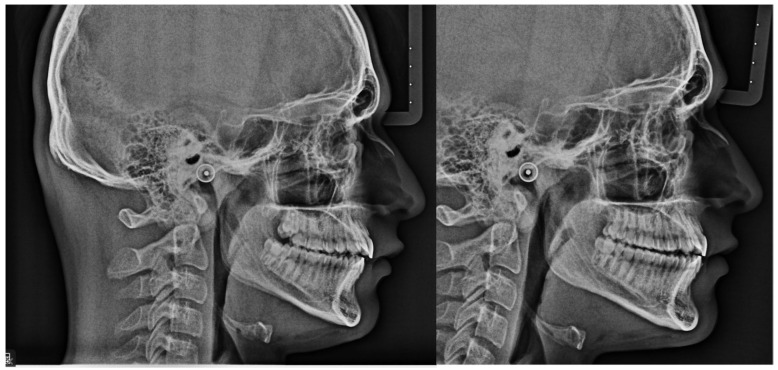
Lateral radiographs before and after treatment with the supragingival miniplate (SGMP)-supported Herbst device.
